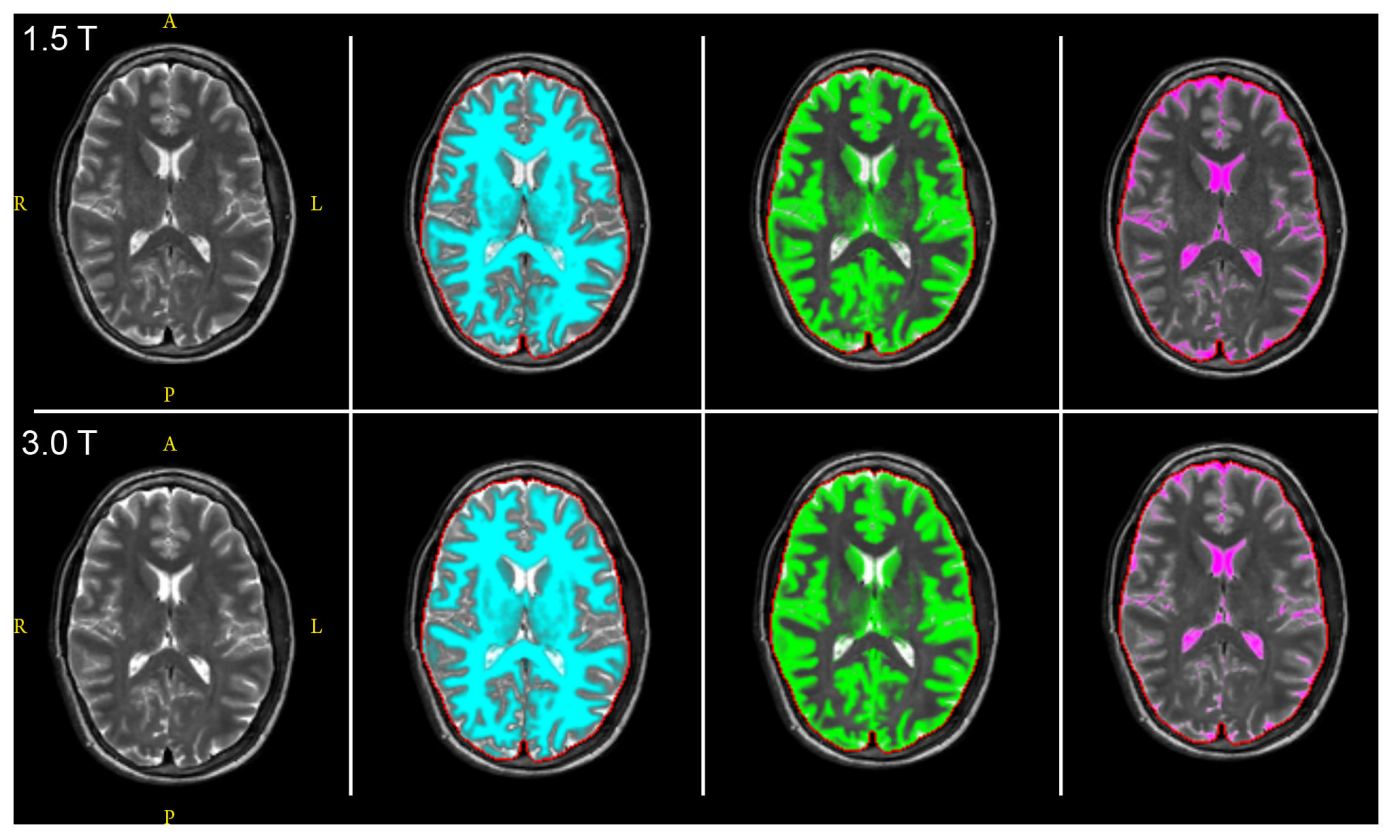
Brain tissue segmentation, of one slice in one healthy subject, at both 1.5 T (top panel) and 3.0 T (lower panel). From left to right: T2-weighted conventional image, white matter (blue), grey matter (green) and CSF (purple). The red lines are the brain intracranial mask calculated automatically by the SyMRI software.
